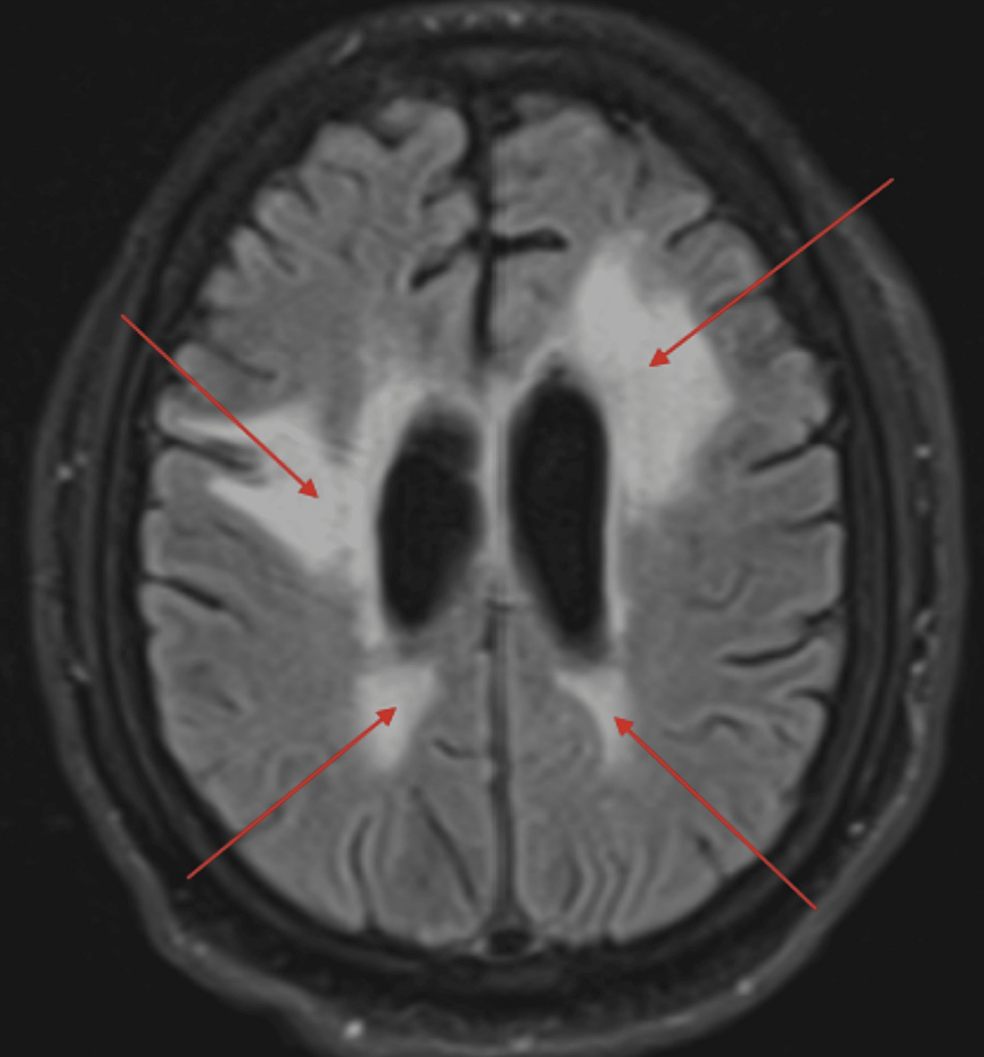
Extensive white matter disease in a patient with VGKC antibody (red arrows) on MRI FLAIR sequence. VGKC: voltage-gated potassium channel; FLAIR: fluid-attenuated inversion recovery.
